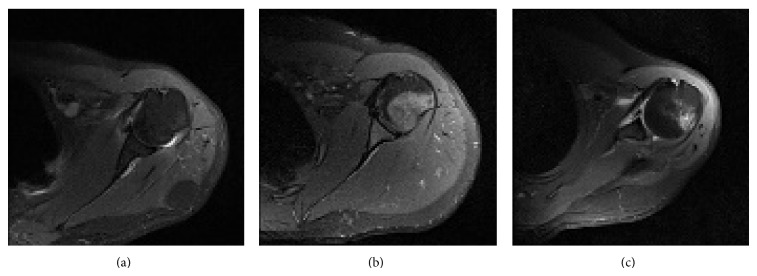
PD weighted axial MR images of left shoulder with (a) normal humeral head, (b) edematous humeral head, and (c) humeral head with Hill-Sachs lesion.
